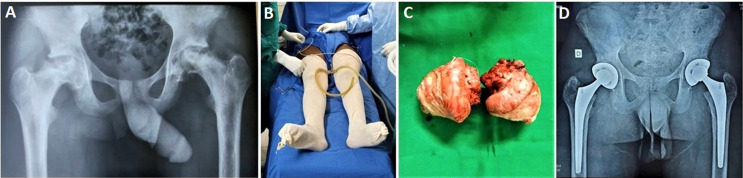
bilateral total hip arthroplasty indicated for end-stage osteoarthritis: A) preoperative X-ray; B) installation on the operating table (double draping); C) enucleation of the two femoral heads; D) postoperative X-ray, Maroua Military Hospital, Cameroon, 2024
